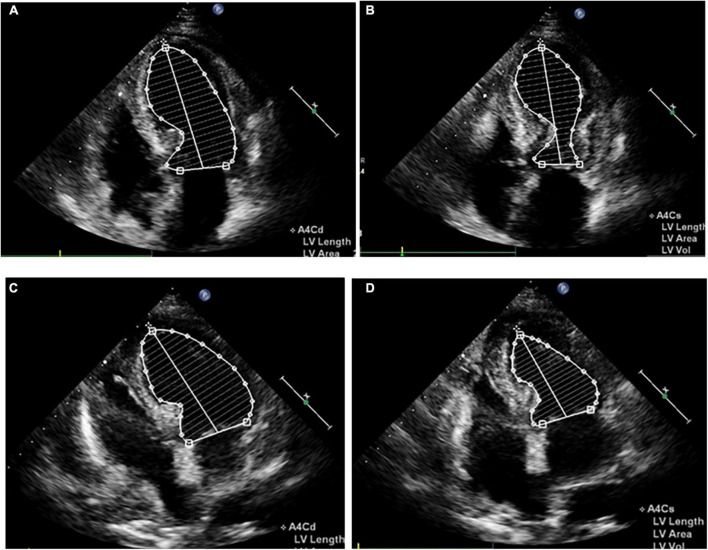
Apical four-chamber view echocardiogram reveals apical dyskinesis and basal to midventricular hyperkinesis (A,B), as well as apex systolic function improvement (C,D). (A,C) Diastole. (B,D) Systole.
